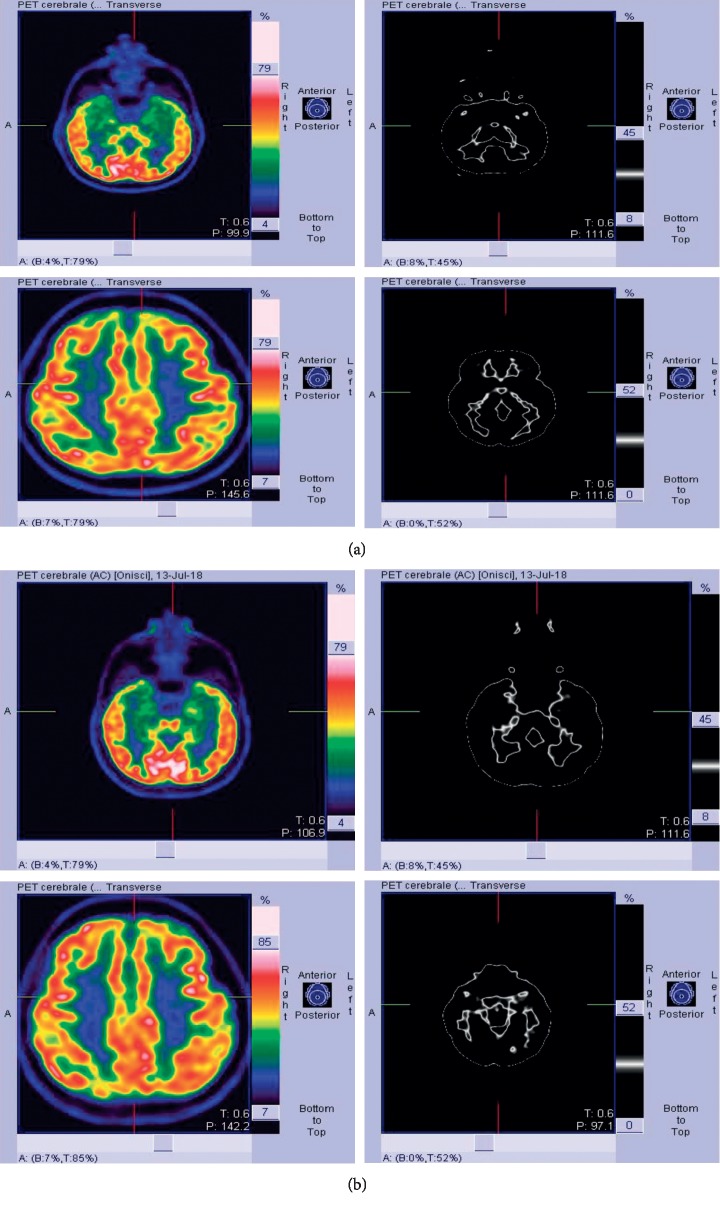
A significant improvement in metabolic uptake at [18F] fluorodeoxyglucose positron emission tomography ([18F]-FDG PET).
